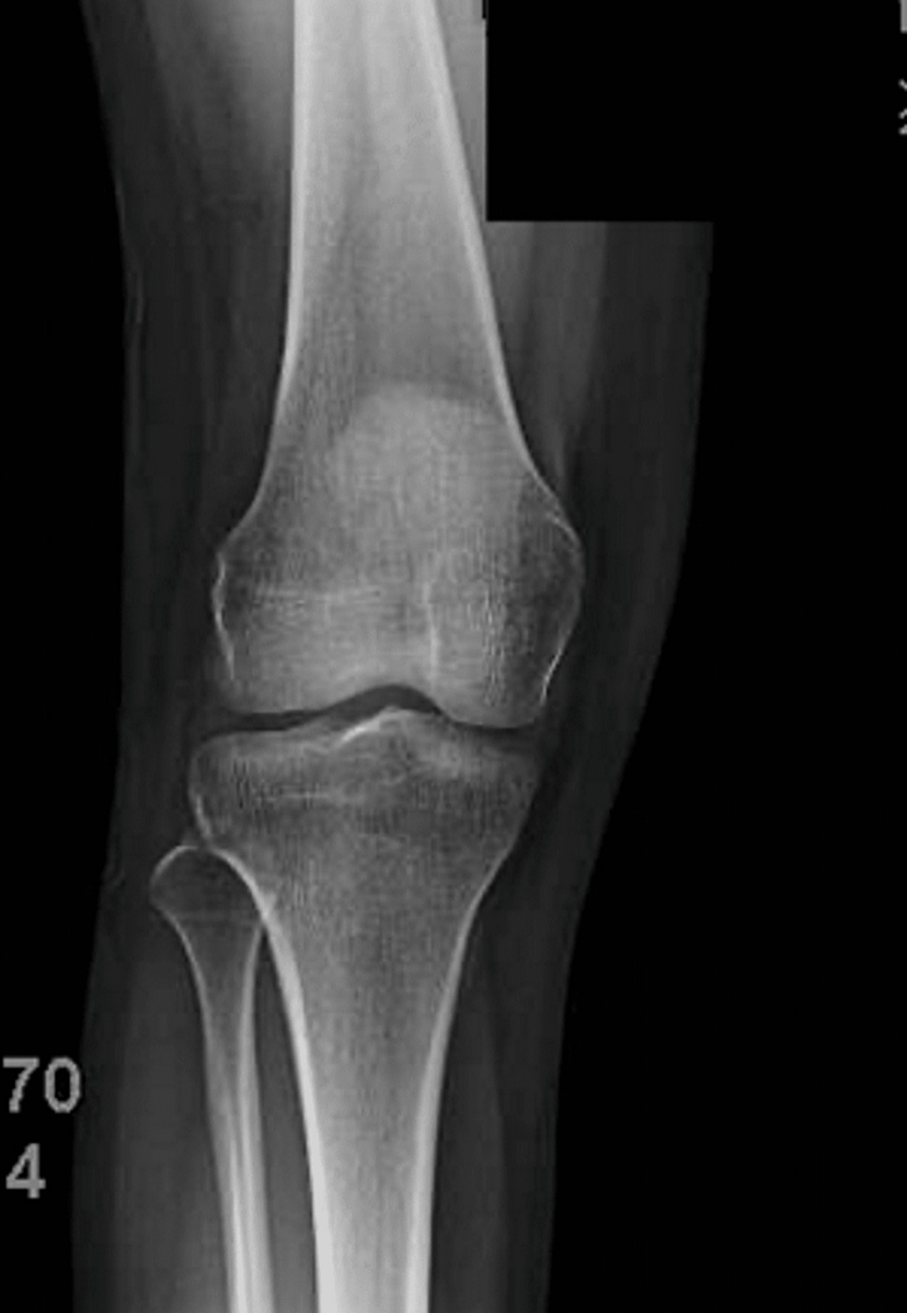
Repeat X-rays taken of the right distal femur, showing the osteochondroma to be completely resolved.
